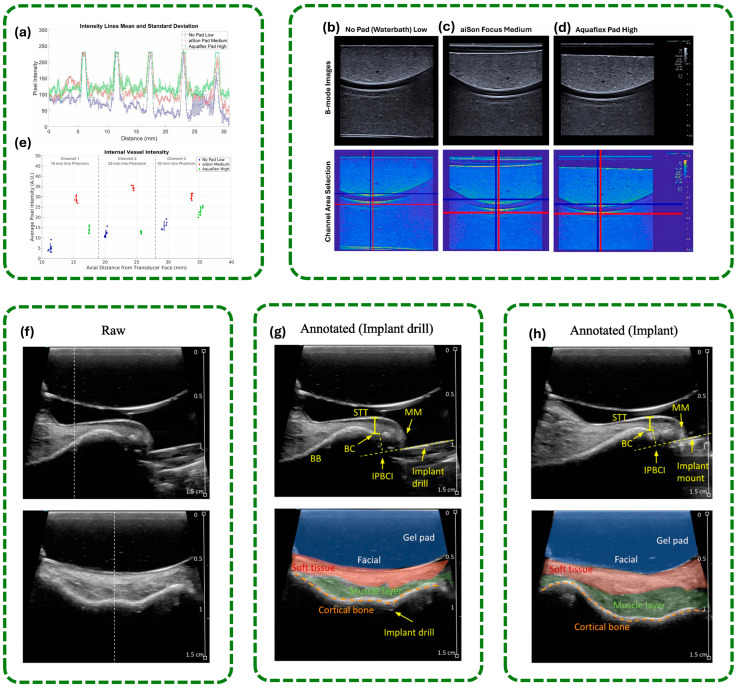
Comparative ultrasound imaging performance of different coupling media. (a) Mean pixel intensity profiles with standard deviation. (b–d) B-mode ultrasound images acquired without pad, with aiSon Focus Medium, and with Aquaflex Pad. (e) Average internal vessel intensity at different depths Reprinted from Elsevier under CC by 4 [105]. (f–h) Raw and annotated ultrasound images showing soft tissue, muscle layer, cortical bone, and dental implant-related structures (implant drill and mount) using the gel pad. Reprinted from Elsevier under CC by 4 [193].
